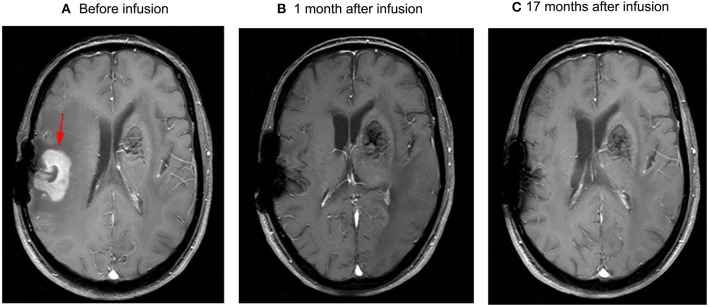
Brain MRI (A) the residual mass on the right side of the post-operative cavity, which was ~26 mm*35 mm*30 mm (arrow) in volume, and the mass disappeared 1 month after infusion (B). No relapse occurred in subsequent follow-up (C).
